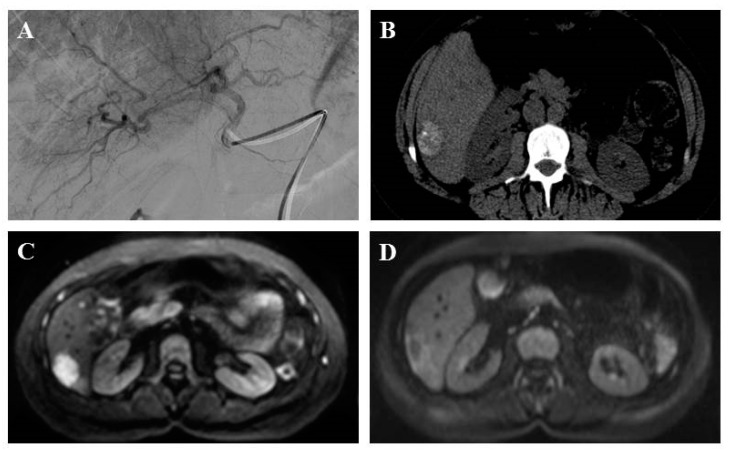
A 51-year-old patient with liver metastasis from pancreatic grade 2 neuroendocrine tumor treated with lipiodol and doxorubicin chemoembolization. Prior to chemoembolization, the arteriography of tumor-feeding branches (2A) and diffusion-weighted axial plane magnetic resonance imaging (b = 1000 s/mm^2^ with restriction of diffusion) (2B) showed a right liver lesion. Axial computed tomography scan without injection showed a major lipiodol uptake after one month (2C). Diffusion-weighted axial plane magnetic resonance imaging (b = 1000 s/mm^2^) showed a complete response at three months (2D).
